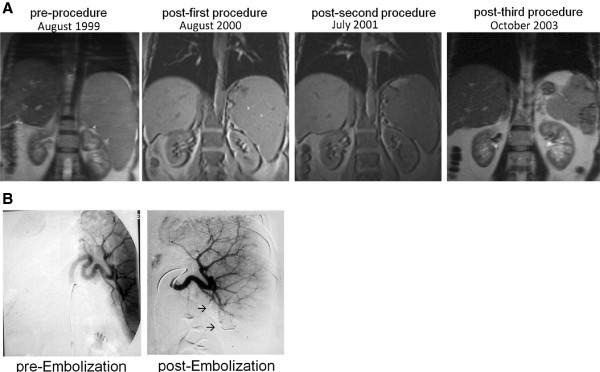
Patient 1: (A) Serial Coronal MRI’s of the abdomen. The splenomegaly is seen to progressively resolve, and there is correction of the displacement of the left kidney over time. (B) Angiograms of the spleen pre and post procedure, demonstrating insertion of radiopaque material in arterial branches (→) and lack of distal opacification.
